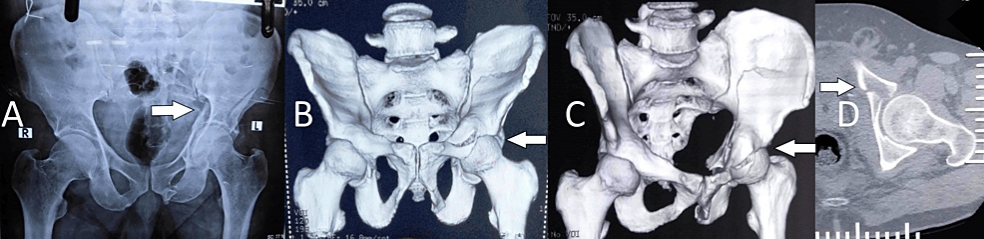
Preoperative radiography and computed tomography images A) Pelvis anterior-posterior (AP) radiograph; B) Anterior-posterior view in three-dimensional reconstruction of computed tomograph (CT); C) Iliac oblique view in three-dimensional reconstruction; D) Axial view in CT scan.
